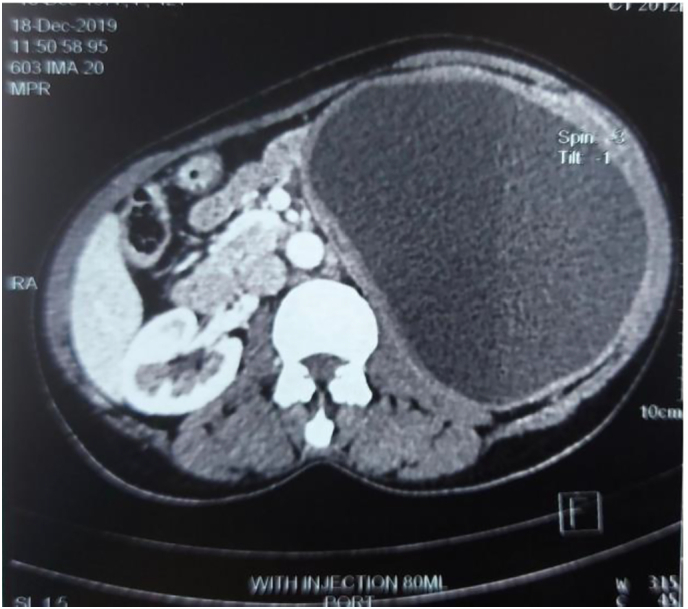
This horizontal section of abdominal CT scan indicates a well-defined cystic mass in the left side of the abdomen.
